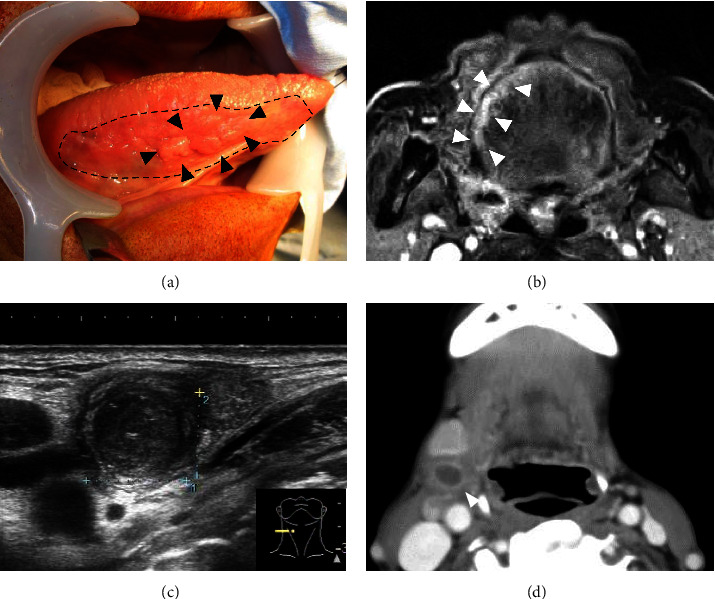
Clinical findings of the patient. (a) A mass lesion of the tongue. An exophytic mass measuring 20 mm in diameter was observed on the right side of the tongue (arrowheads), which was surrounded by a flat white leukoplakia-like lesion (dotted line). (b) Magnetic resonance imaging with gadolinium contrast. Enhancement of the lesion (white arrowheads) at the right edge of the tongue is observed. The tumor measured approximately 4 mm in thickness. (c) Ultrasound examination. An enlarged lymph node was detected in the superior internal jugular node area. The node was round and showed internal heterogeneous echoes; therefore, it was suspected as a metastasis. (d) Computed tomography with contrast. An enlarged lymph node is seen on the right side of the neck, containing a low-density area within and an enhancing edge, similar to the characteristic findings of lymph node metastasis from squamous cell carcinomas.
